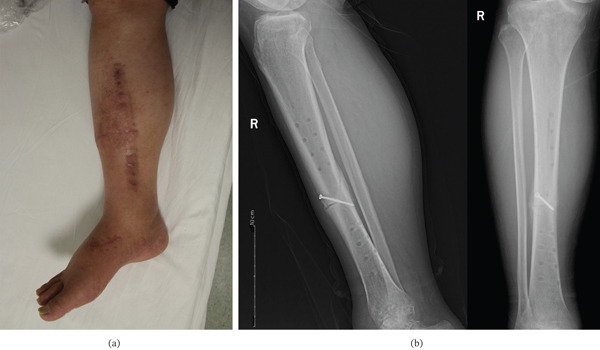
(a) The modified external fixation removed at the 3‐month follow‐up. (b) Posteroanterior radiographs at the 12‐week (3‐month) follow‐up after device removal: lateral view (left) and anteroposterior view (right) demonstrating healed fracture with bridging callus across all four cortices. The modified RUST score was 13, confirming clinical union.
